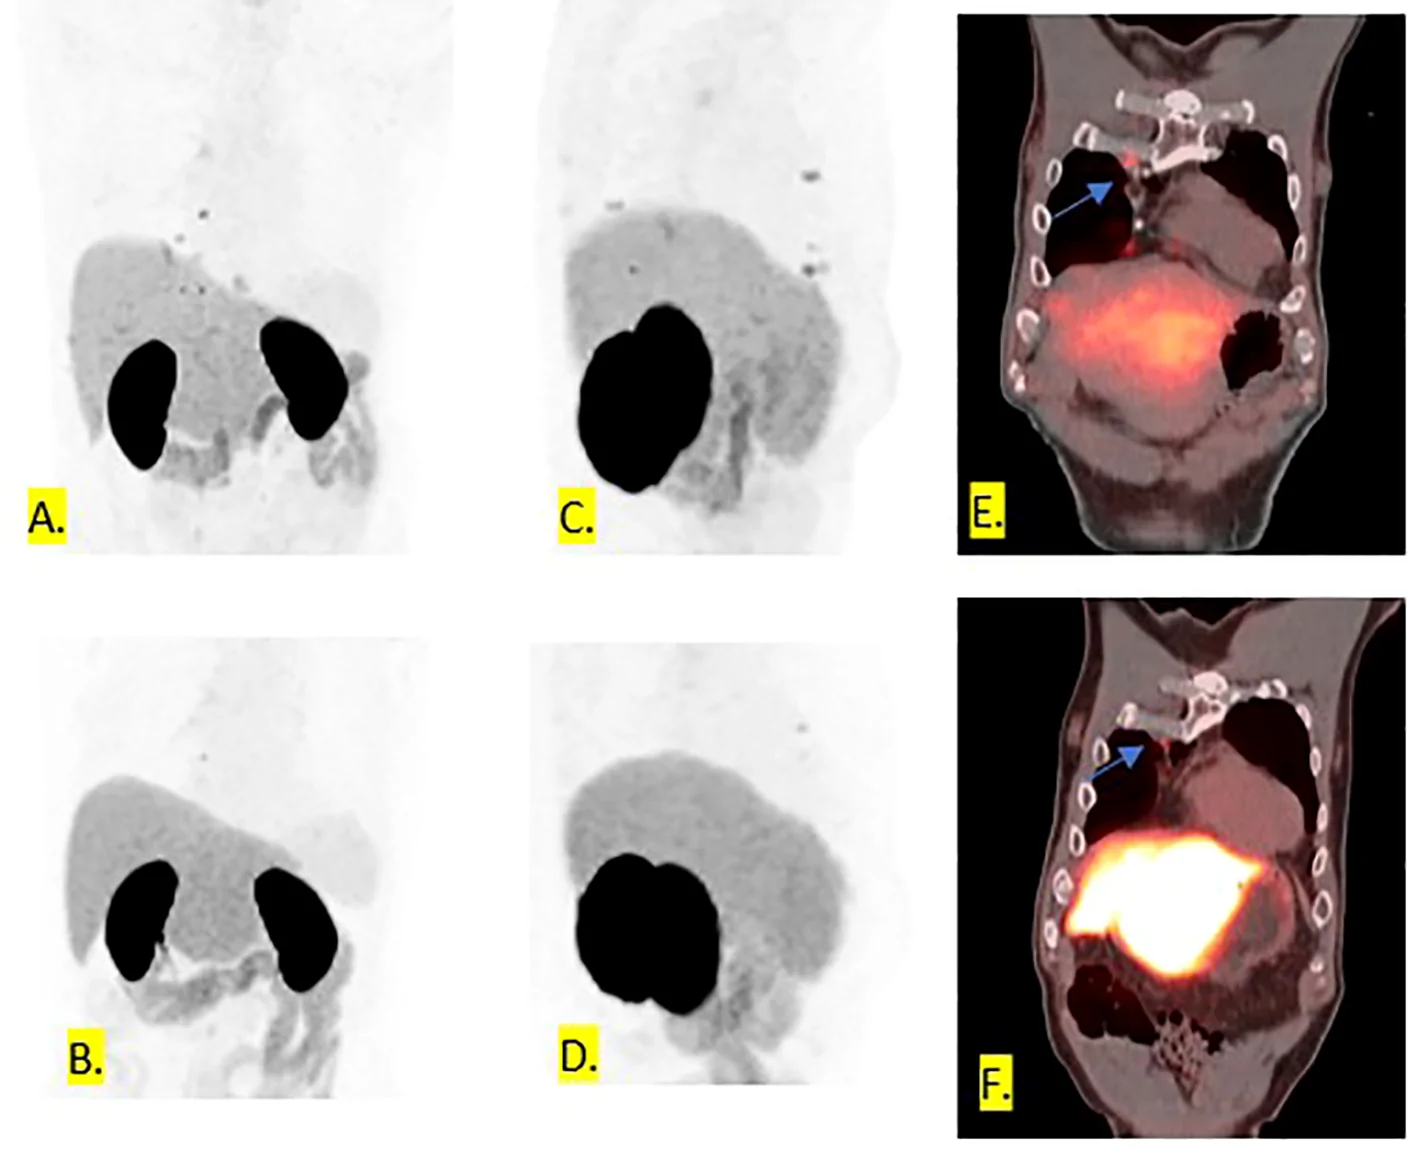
Pre- and post-treatment psma-pet/ct imaging in prostate adenocarcinoma. All images were obtained using the same scanner. Pre-treatment scans were performed 1 year apart pre and post IDCT. (A) Coronal MIP image showing multiple PSMA-avid thoracic lymph nodes prior to IDCT, at which time PSA was 78 ng/ml. (B) Coronal MIP image obtained two months after the third IDCT injection, demonstrating resolution of all lesions except one. (C) Sagittal MIP image prior to IDCT. (D) Sagittal MIP image following IDCT. (E) Coronal fusion image prior to treatment, with a standardized uptake value (SUV) of 3.1 at the pointed internal mammary lymph node mass. (F) Coronal fusion image post-treatment, showing reduced SUV of 1.5 of the one remaining internal mammary lymph node (pointed).
